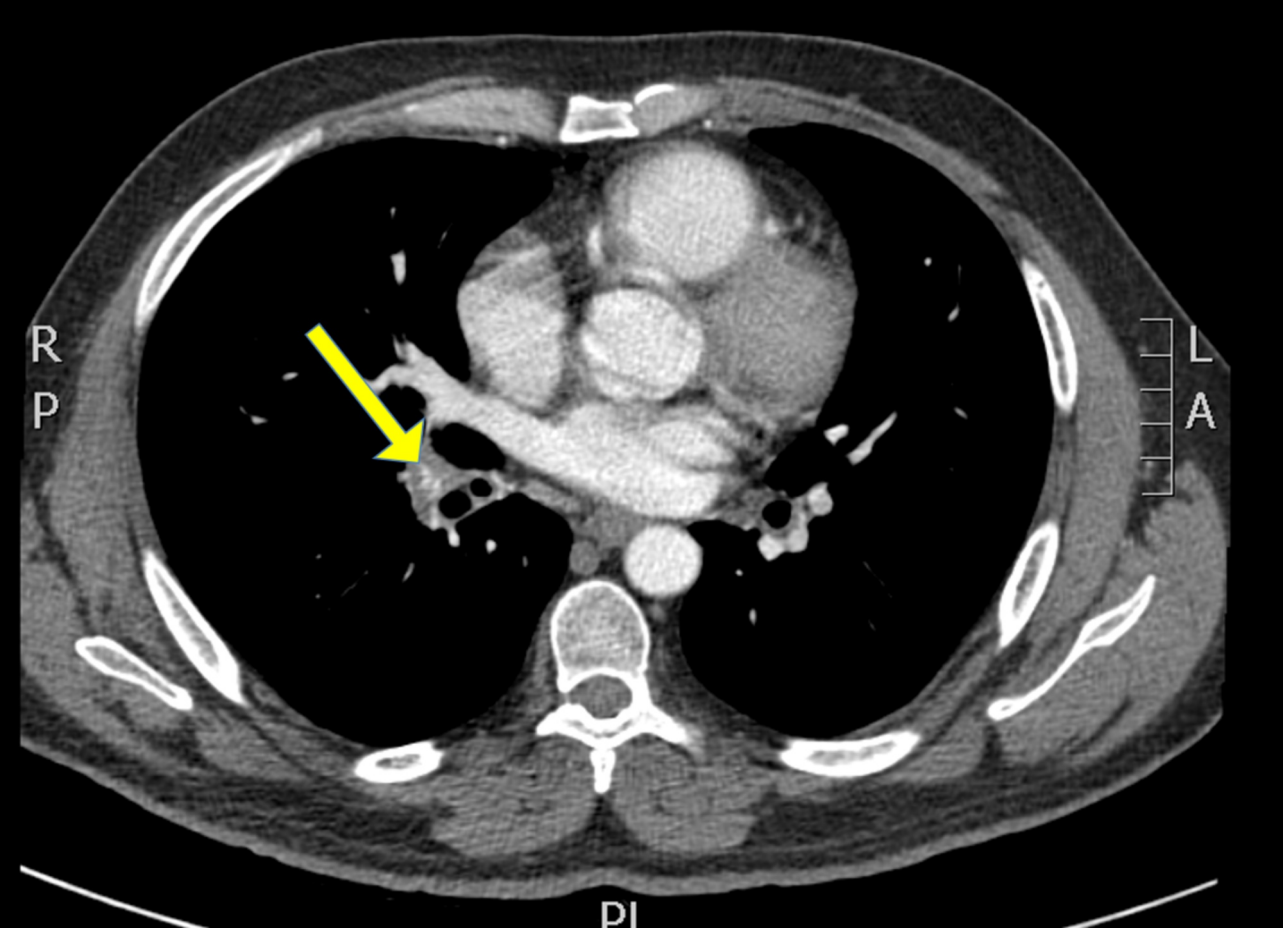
Chest CT showing significant decrease in the pulmonary artery size CT, computed tomography
